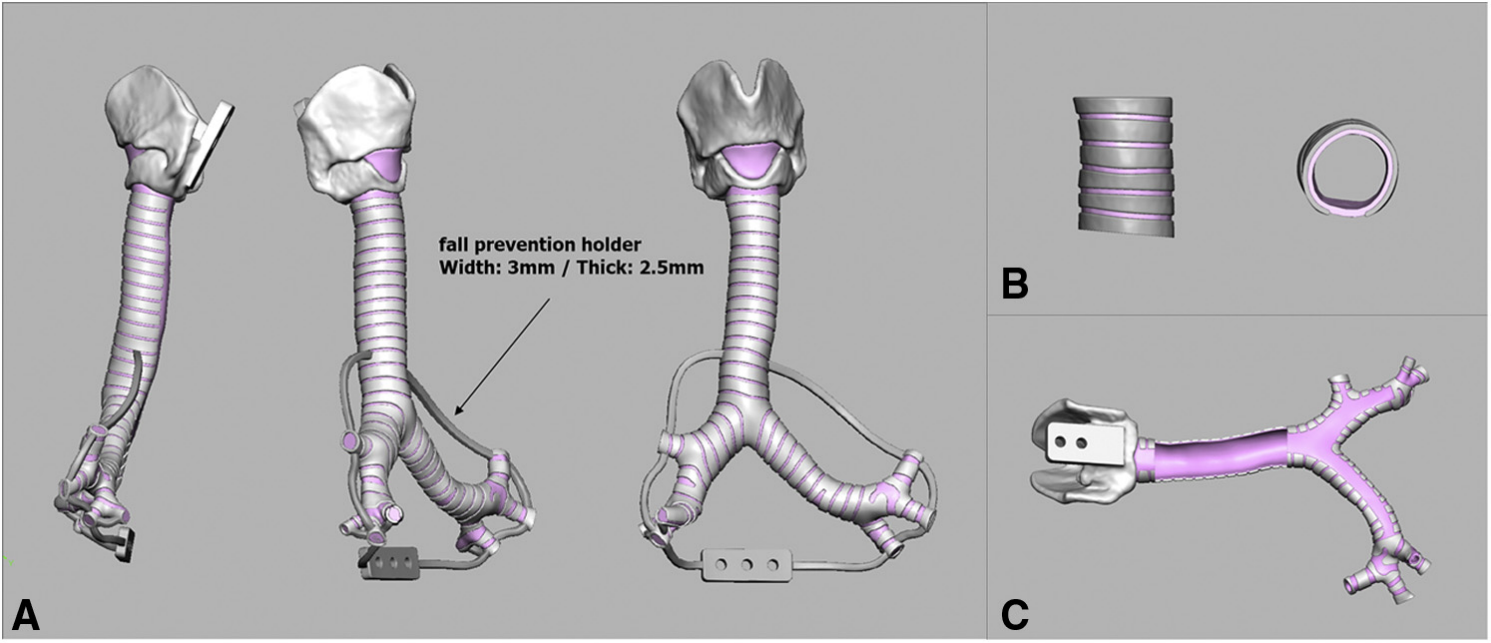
Design of a 3-dimensional airway model using data obtained during chest computed tomography. A, An overall view. A fall prevention holder was attached to avoid part of the model falling during procedure. B, A magnified image and an axial view. C, A back view showing the area of membranous portion. Note that white and pink areas were recognized separately, and 2 different urethans were utilized to represent airway anatomy.
